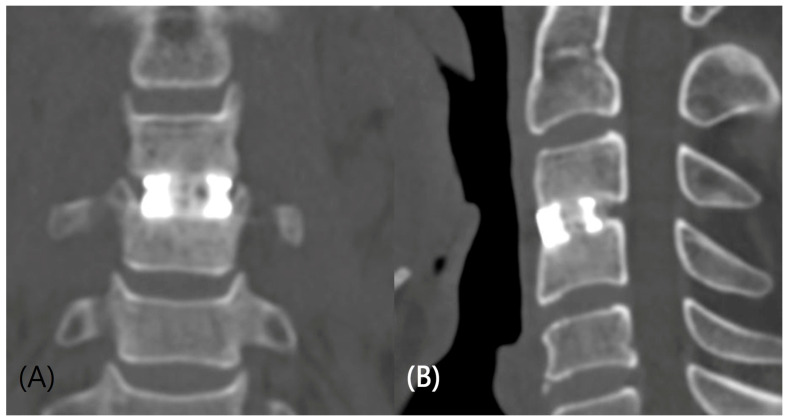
Radiographic assessment of fusion. Coronal (A) and sagittal (B) reconstructed 3D-CT images at 12 months postoperatively, demonstrating solid bone bridging and complete fusion across the operated disc space in a patient who received i-Factor™ Bone Graft. Original images from this study cohort. Patient: a 46-year-old male who underwent single-level C3−4 ACDF for with C3−4 disc herniation and compressive myelopathy refractory to conservative treatment.
